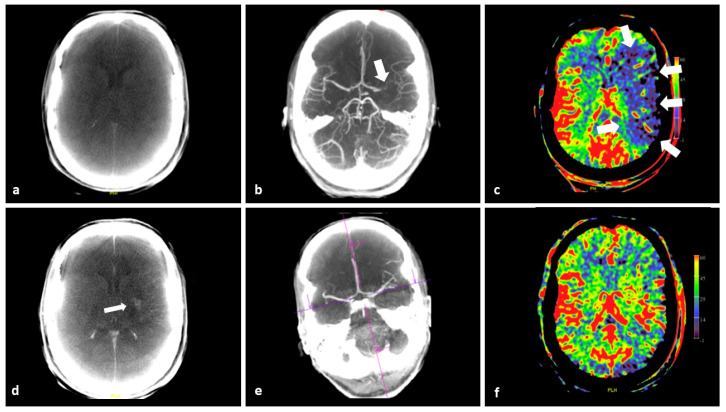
FD CT imaging in a 44-year-old female with AIS due to LVO, referred for EVT. (a) Pre intervention axial MIP reformation of the mask run demonstrates no hemorrhage. (b) Pre intervention axial MIP reformation shows occlusion at the level of M1-segment of the left MCA (arrow). (c) PBV MIP reformation features a perfusion deficit in the left MCA supply area (arrows). (d) Post EVT axial mask run MIP reformation demonstrates small area of contrast pooling in the left lentiform nucleus and the revascularized MCA territory (arrow). (e) Post EVT axial fill run MIP reformation confirms recanalization. (f) Post EVT axial PBV MIP reformation shows restoration of CBF with (almost) symmetrical PBV map.
